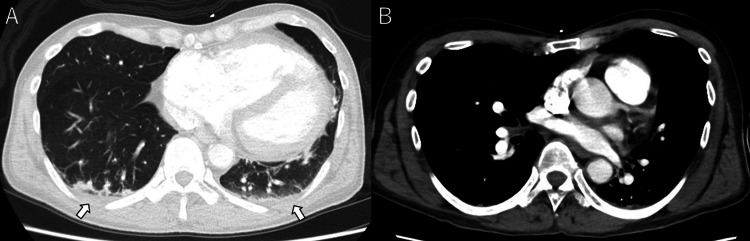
Chest computed tomography findings at admission (A) Non-contrast chest computed tomography (CT) revealed bilateral dorsal infiltrates, suggestive of pneumonia (arrow). (B) Contrast-enhanced chest CT showed no detectable thrombus in the pulmonary arteries.
